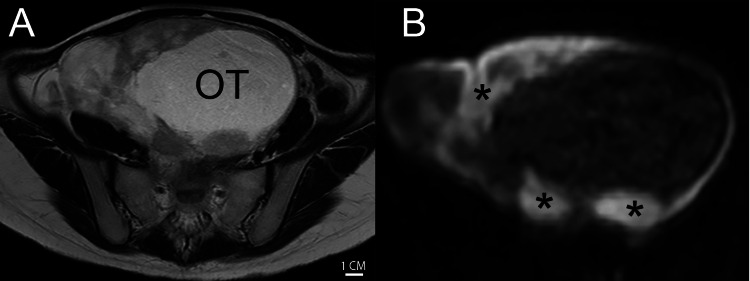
Preoperative MRI of the pelvis. (A) T2-weighted axial view, demonstrating a 16×15 cm mixed cystic-solid pattern mass with a dominant cystic part. OT, ovarian tumor. Scale bar, 1 cm. (B) Diffusion-weighted axial view, demonstrating diffusion restriction in peripheral solid parts (*).
